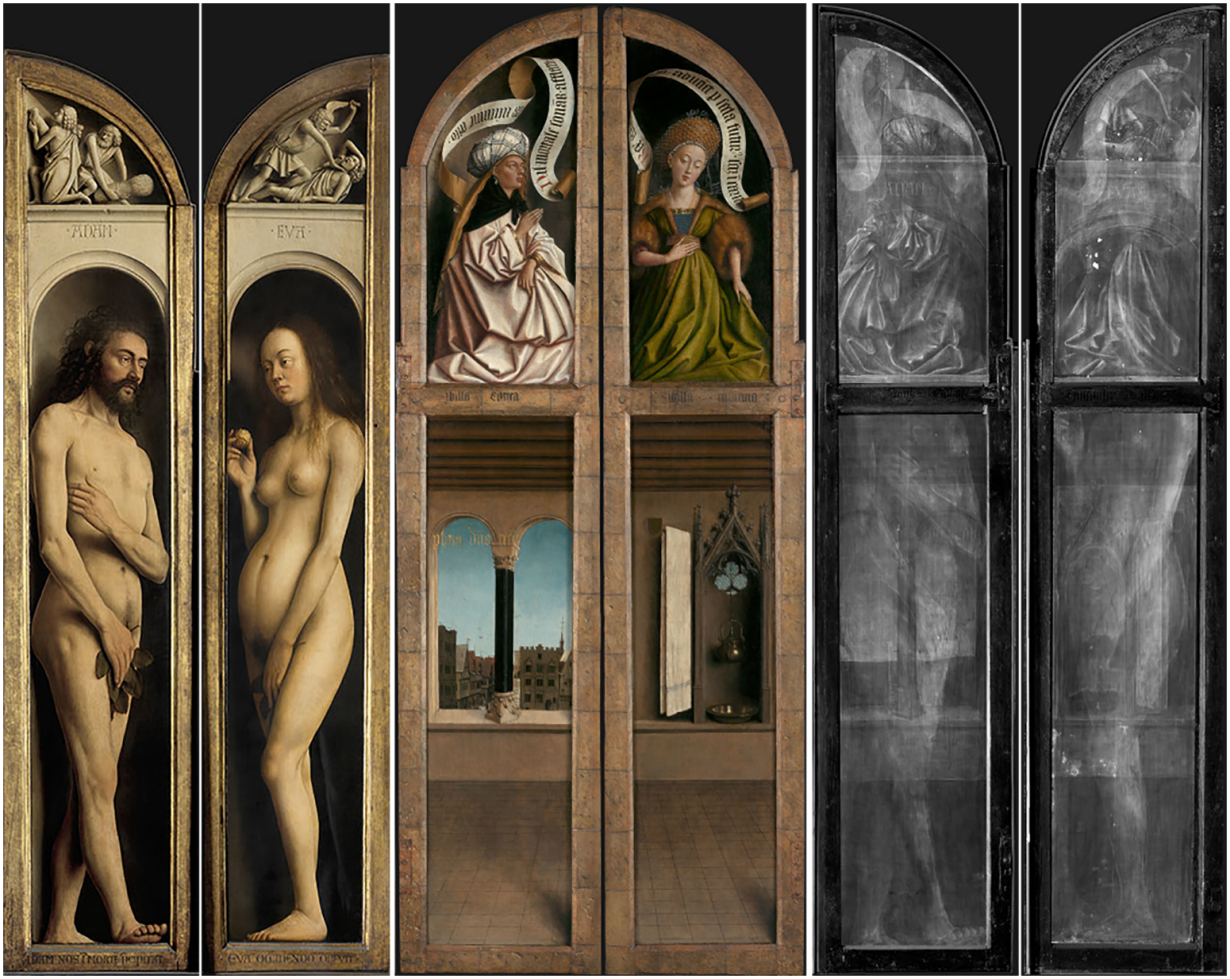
The two double-sided panels from the *Ghent Altarpiece*. Interior view of shutters (left, before conservation), exterior view of shutters (center, after conservation), and corresponding x-ray images for each panel (right, acquired before conservation), which include the combined contributions from both sides of each panel. Note that because x-ray imaging captures both sides by penetrating through the panel, whereas the panel has to be turned over to photograph its backside in visible light, the x-ray images superpose the mirror image of one side and the nonmirrored image of the other side. [X-ray image in this figure, and for details in further figures, are used with permission of the copyright holder, Saint-Bavo’s Cathedral (Art in Flanders; www.lukasweb.be). Photo credit: KIK-IRPA.]
